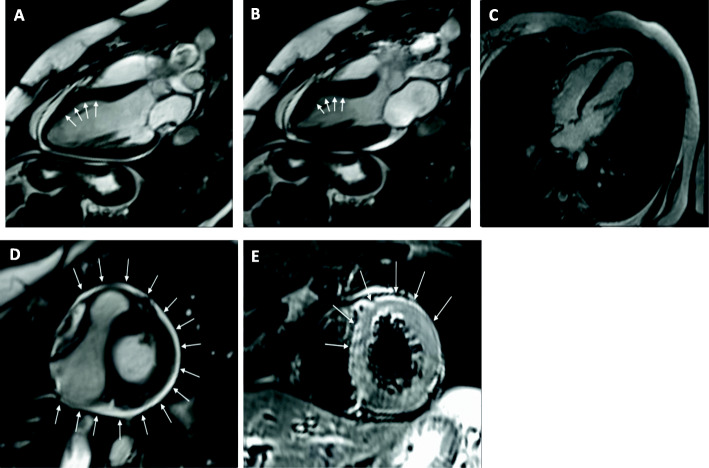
Cine cardiac magnetic resonance images showing a 3-chamber view of the left ventricle in end diastole (a) and in end systole (b) demonstrating a circumferential mid-ventricle hypokinesia with greater intensity in the anteroseptal segment (arrows). (c) image shows late gadolinium enhancement sequence showing a 4-chamber view of the heart without any sign of myocardial fibrosis. (d) Cine cardiac magnetic resonance sequence demonstrating diffuse pericardium effusion of moderate intensity (arrows). (e) Short-tau inversion-recovery (STIR) sequence, a T2-weighted sequence used to assess myocardial edema, demonstrating the presence of hypersignal in the middle segments of the anterior, septal and lateral walls (arrows) which indicates the presence of myocardial edema in these segments
